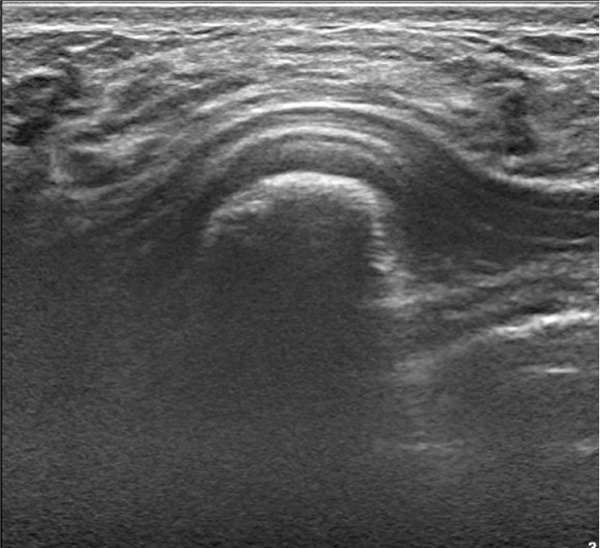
Right breast ultrasound demonstrating abnormal mass.
